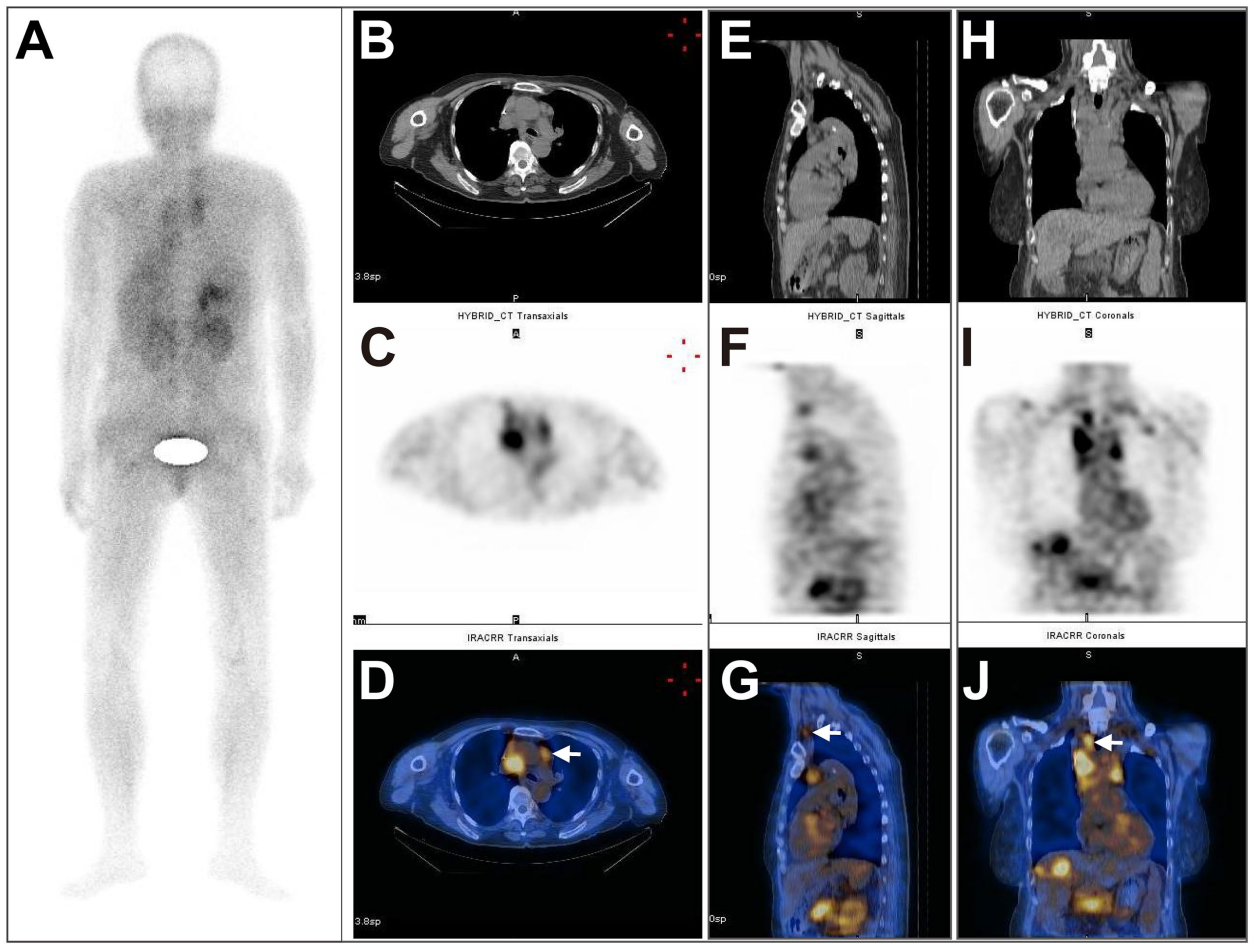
A 66-year-old postoperative female patient with sigmoid colon adenocarcinoma (pT3N0M1, stage II) underwent ^99m^Tc-FAPI-04 SPECT/CT and CECT examinations. The whole-body MIP image (A). The axial (B), coronal (E), and sagittal (H) CT images, the axial (C), coronal (F), and sagittal (I) SPECT images, and the corresponding fused ^99m^Tc-FAPI-04 SPECT/CT images (D,G,J), where a high accumulation of ^99m^Tc-FAPI-04 occurred not only in the lymph nodes in the neck, supraclavicular fossa, mediastinum, hepatic portal area, and retroperitoneum but also in the liver and right adrenal gland. The white arrow indicates high ^99m^Tc-FAPI-04 uptake in metastatic lesions.
